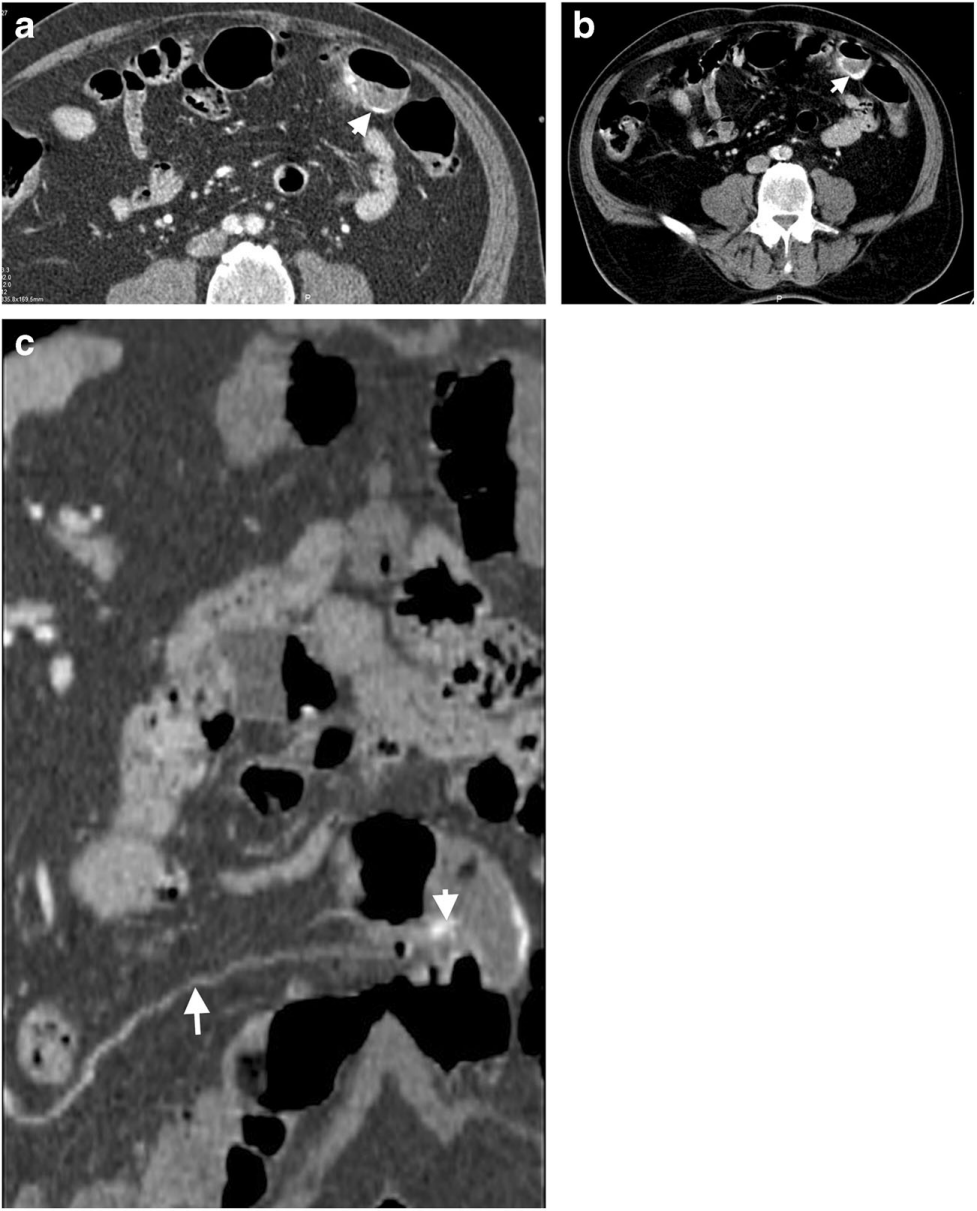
Bleeding diverticulitis in a 70-year-old male. a Axial contrast-enhanced CT of a 70-year-old man without oral contrast agent administration reveals intraluminal high attenuating contrast agent leakage (*arrowhead*) in transverse colon. b Axial contrast- enhanced CT at venous phase demonstrates increased contrast amount in the lumen (*arrow*) indicating active hemorrhage. c Oblique reformatted CT image reveals feeding artery (*arrow*) of the diverticulitis and contrast extravasation (*arrowhead*)
